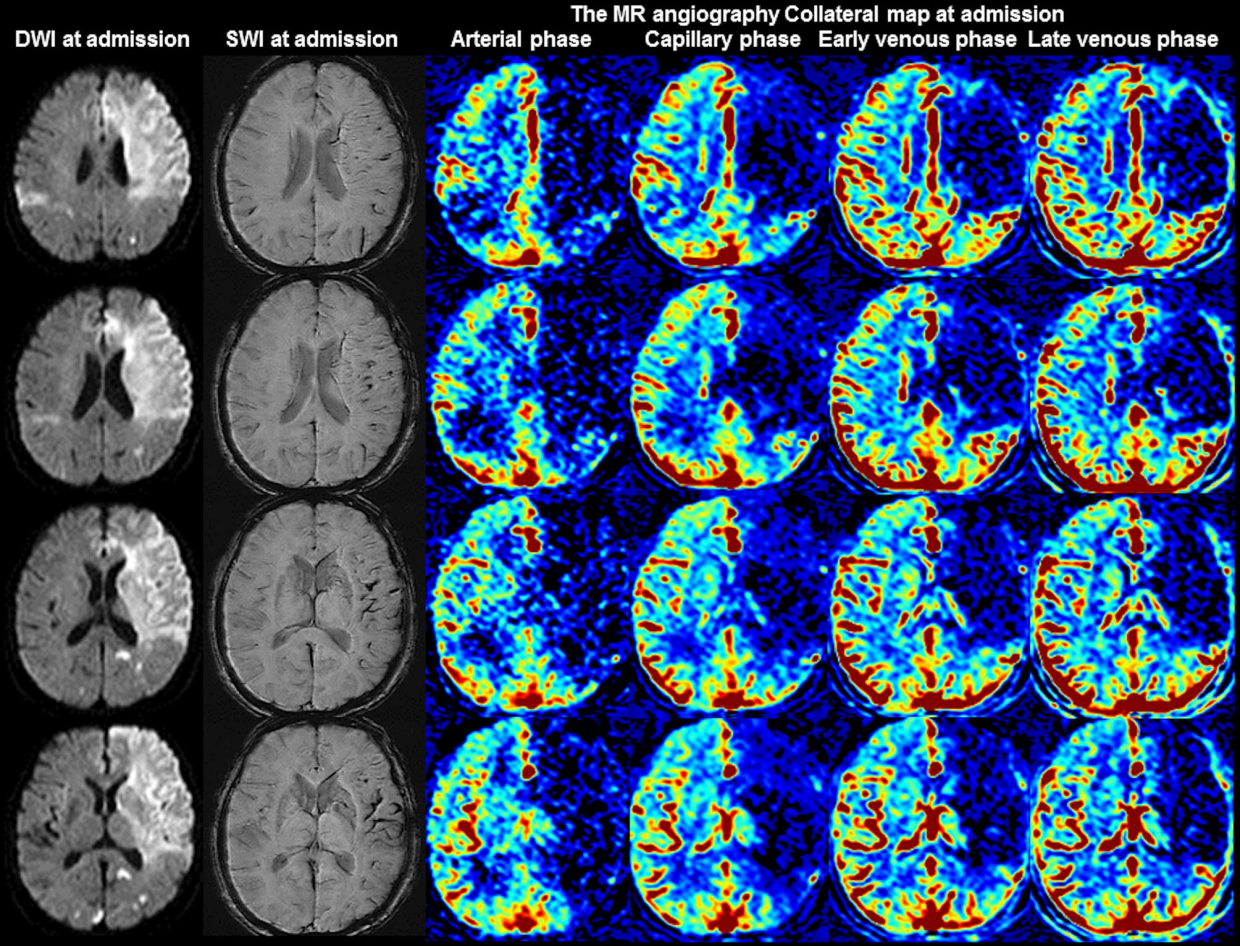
Images of a 72-year-old woman with occlusion of the left internal carotid and middle cerebral arteries. The premorbid modified Rankin scale (mRS) score of this patient was 0, and the National Institutes of Health Stroke Scale score at admission was 20. The diffusion-weighted imaging (DWI), susceptibility-weighted imaging (SWI), and multiphase MR angiography collateral map at 5 h and 25 min after symptom onset are shown. DWI shows acute infarct signals in both cerebral hemispheres. Acute infarction in the right cerebral hemisphere was considered to indicate another embolic infarction. SWI shows very prominent cortical and medullary veins in the left middle cerebral artery territory, representing very poor collateral status. The collateral-perfusion delay of more than one-half of the left middle cerebral artery territory persisted until the late venous phase on the MR angiography collateral map (MR acute ischemic stroke collateral score of 0: very poor collateral perfusion defined as collateral-perfusion delay/defect more than one-half of the ischemic MCA territory in the late venous phase regardless of perfusion status at previous phases). The patient underwent conservative treatment and died of malignant infarction.
